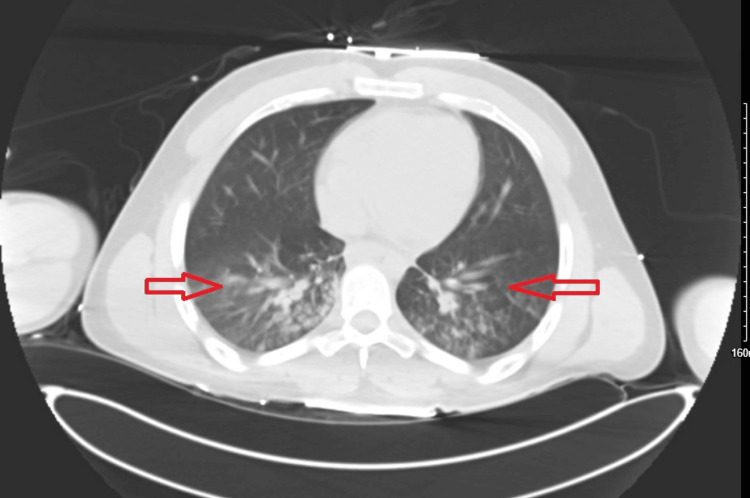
Initial computed tomography showing bilateral opacities and infiltrates suspicious for pneumonia (red arrows).
